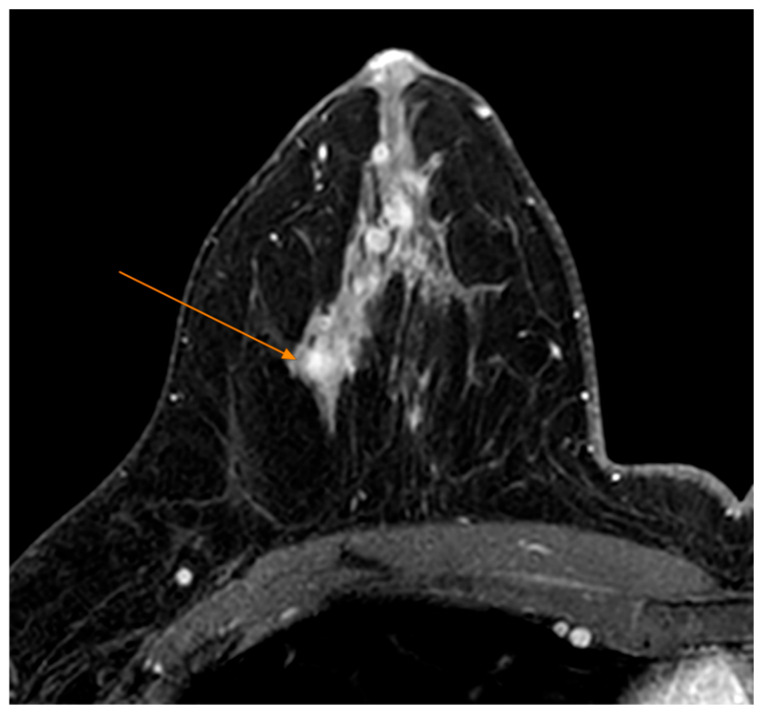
MRI Examination—T1 FS Sequence Post IV Contrast—Nodular mass-like contrast enhancement, located deep in the right breast gland, external quadrant (arrow).
